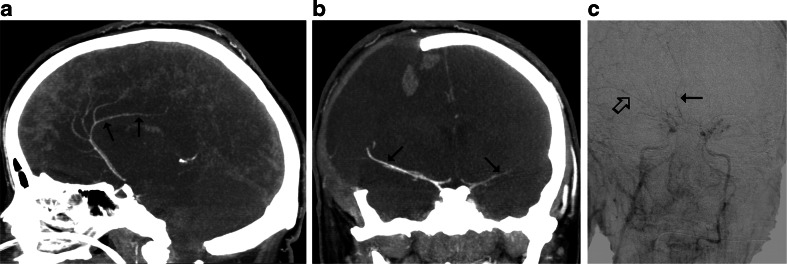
CTA findings in a 22-year-old woman (patient no. 42) with a brain stem ischemic stroke and a right sided craniectomy who presented with signs of BD on clinical examination. a Ten millimeter MIP in sagittal plane. CTA shows opacification of the right pericallosal artery (thin arrows); b Ten millimeter MIP in coronal plane. CTA shows opacification of the M1 segments of the MCAs (*thin arrows*). These findings exclude the diagnosis of BD according to the 10- and 7-point scales but confirm BD according to the 4-point scale. c Catheter angiography from the aortic arch performed 1 h later revealed delayed, residual filling of the M1 segment of the right MCA (*thick arrow*) and A2 segment of the right ACA (*thin arrow*) that occurred 32 s after injection. This result was interpreted as stasis filling consistent with the diagnosis of BD
